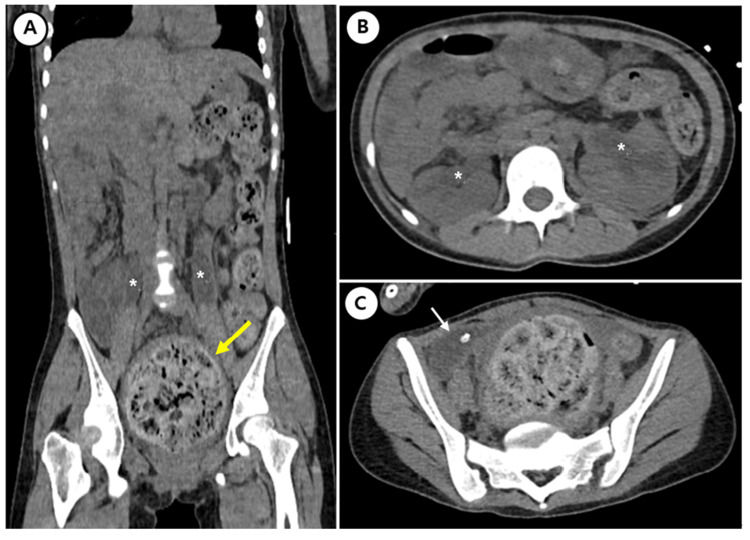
Non-enhanced computed tomography of the abdomen (A) Computed tomography of the abdomen (coronal plane) reveals a large amount of stool retention (yellow arrow) and its upstream dilated distal ureter (*). (B) Computed tomography of the abdomen (axial plane) shows both hydronephroses (*). (C) Computed tomography of the abdomen (axial plane) indicates a collapsed bladder due to a distended rectum (white arrow).
